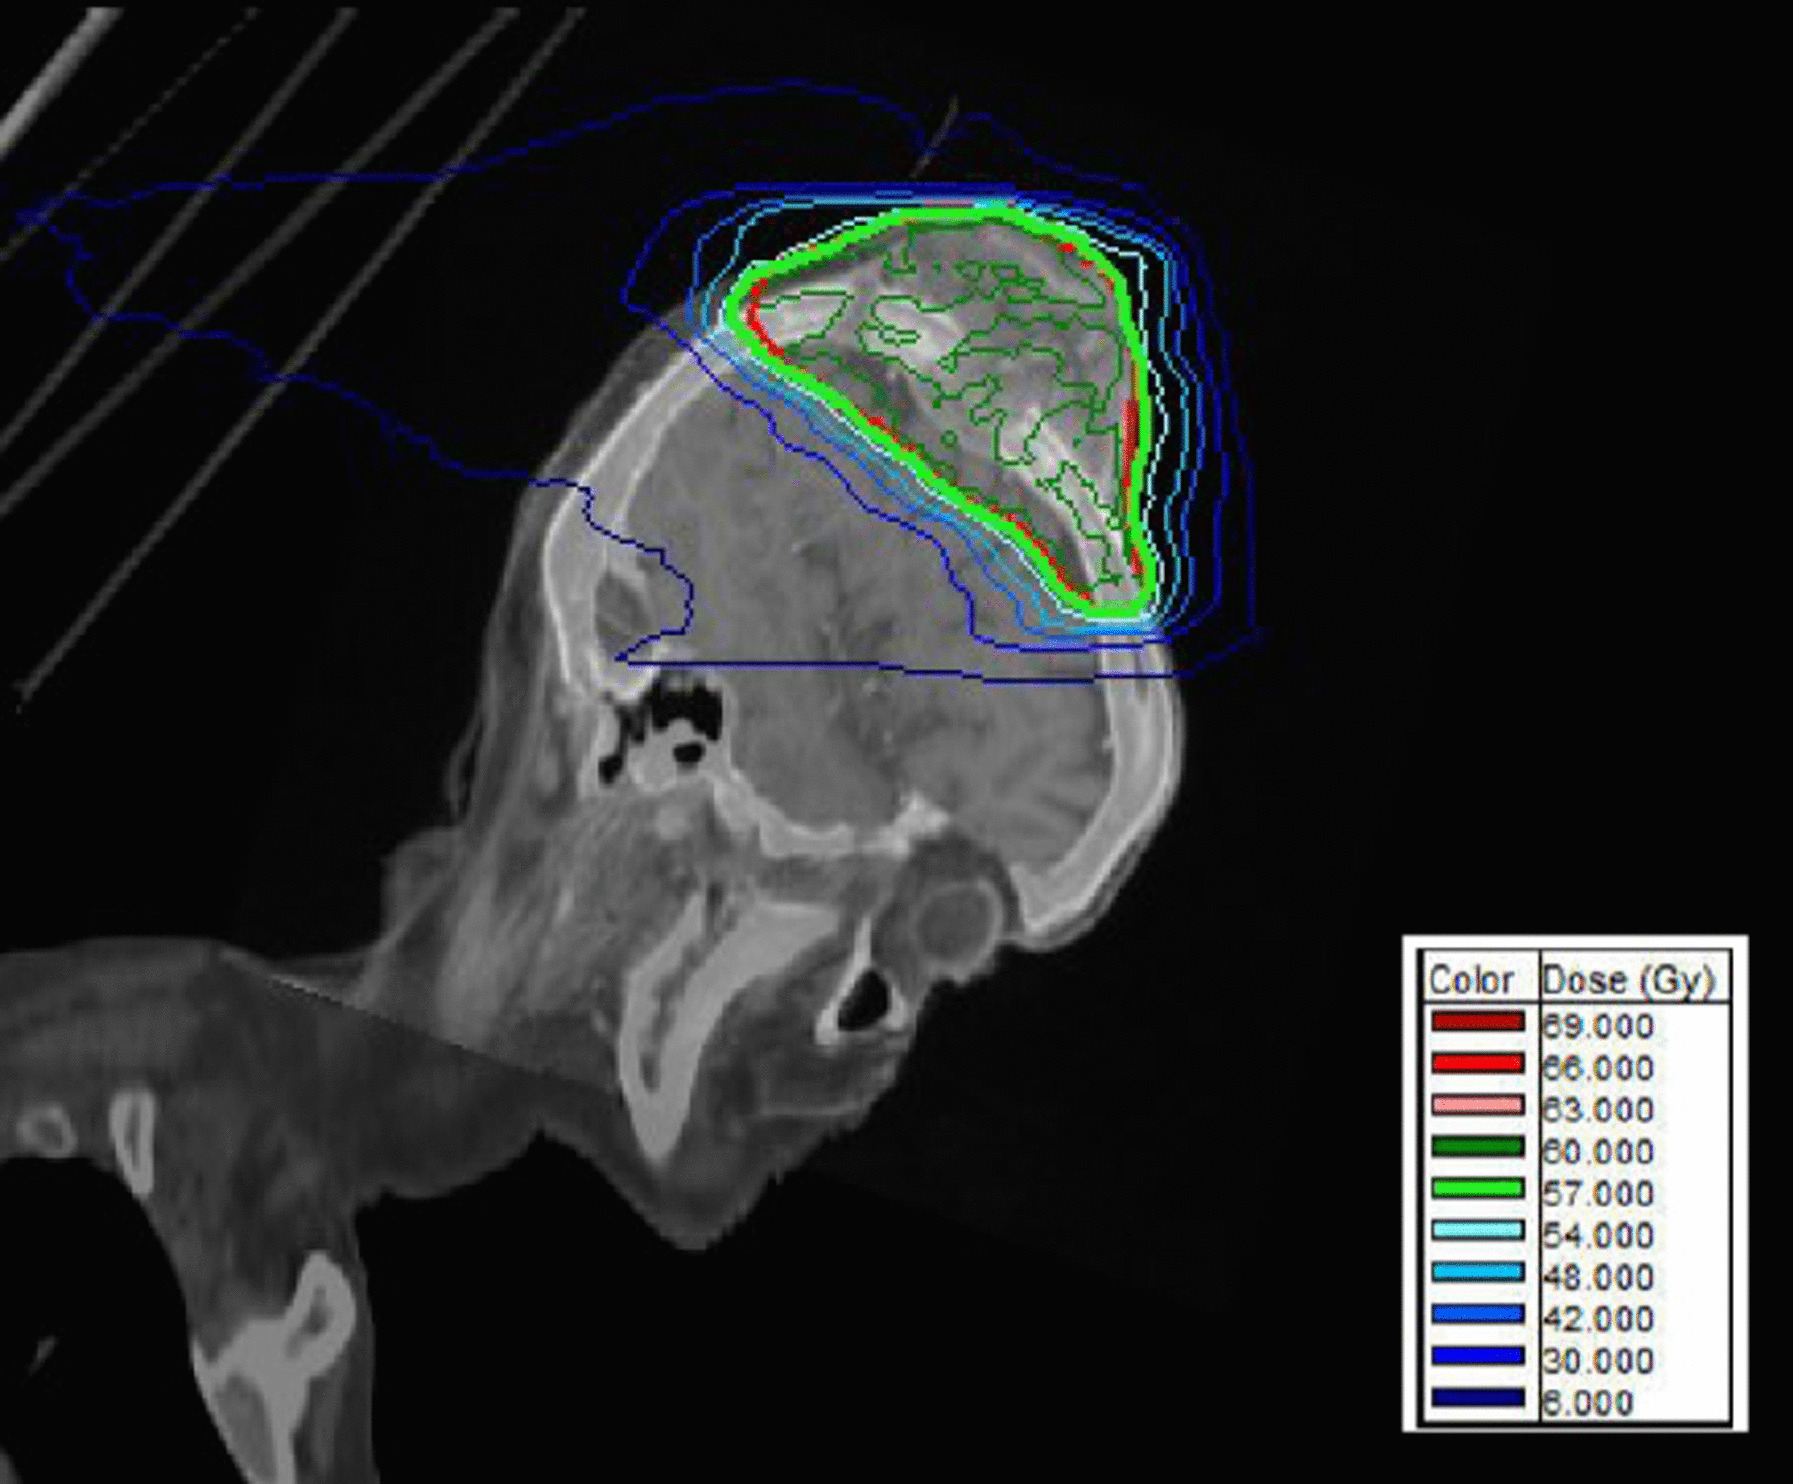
Sagittal view of planning computed tomography images in fusion with MRI. The planning target volume (thick red line) covered the gross tumor volume plus an appropriate margin and was treated with a total dose of 60 Gy at 2 Gy per daily fraction over 6 weeks (BED _10_ 72 Gy). Radiation dose distribution represented by radiation isodose lines: The thick red line represents the planning target volume and the thick green line represents the 57 Gy isodose line (that is 95% isodose, encompassing planning target volume). One cm thick bolus material was used to ensure sufficient surface dose.
